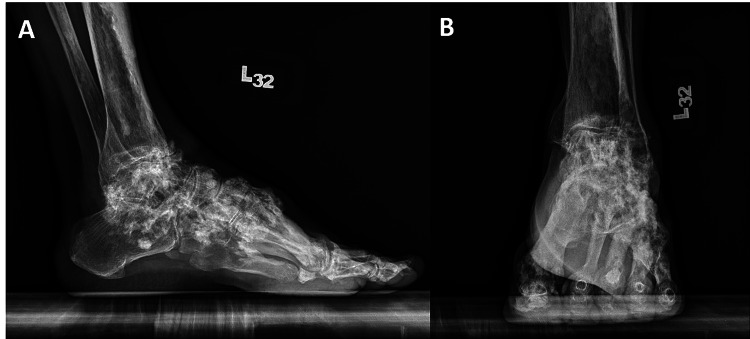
Preoperative weight-bearing radiographs of the left ankle. The images show (A) lateral view and (B) anteroposterior view. A classic “candle-like flowing wax” appearance can be observed, consistent with melorheostosis.
